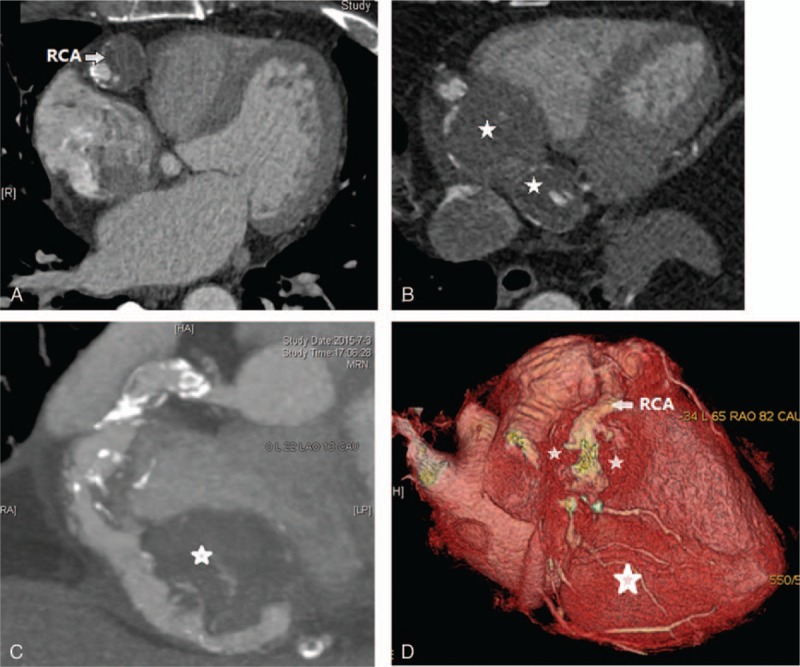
Coronary CTA confirmed that the “mass” were the giant aneurysms of RCA with thrombosis (A, B). The maximum diameters were 25 and 38 mm, respectively, which caused the compression of the right atrium (C). CTA = computed tomography angiography, RCA = right coronary artery.
